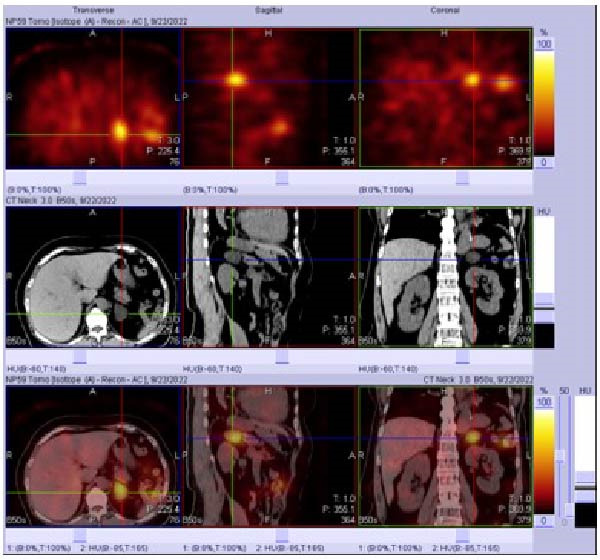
NP‐59 SPECT/CT demonstrating a left‐sided functioning adrenal adenoma. Fusion SPECT/CT imaging revealed focal accumulation of NP‐59 radiotracer in the left adrenal gland, consistent with a hyperfunctioning adrenal cortical adenoma.
